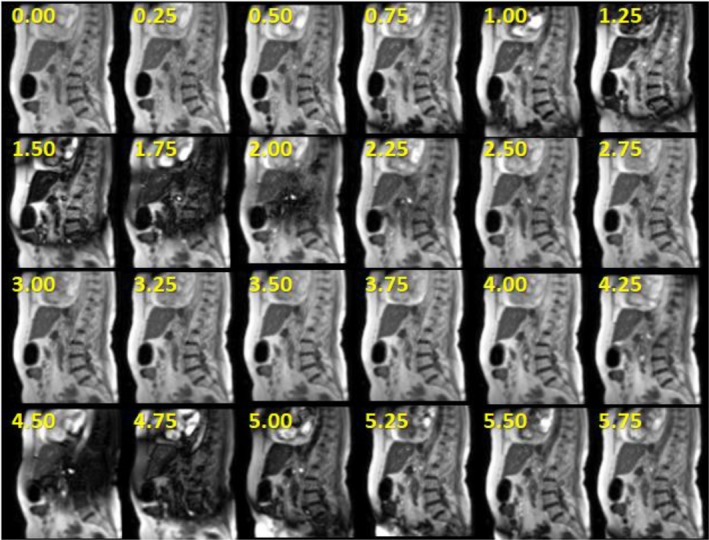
EMI‐related (moving metal) dephasing artifacts that occurred during gantry angle rotation from 300^0^ to 320^0^ in 76‐year‐old female patient receiving adaptive MR‐IGRT for pancreatic cancer on the MRI‐Linac. The numbers represent the time in seconds corresponding to each frame during the gantry rotation. The cine images used 2D sagittal TrueFISP cine (TE/TR: 0.91/2.10 ms, 60^0^, GRAPPA 2, 3.5 × 3.5 × 7 mm, 1351 Hz/pixel, 2 averages, 4 frames/s). Radiation delivery is paused during gantry rotation. Therefore, there is no degradation in treatment accuracy.
